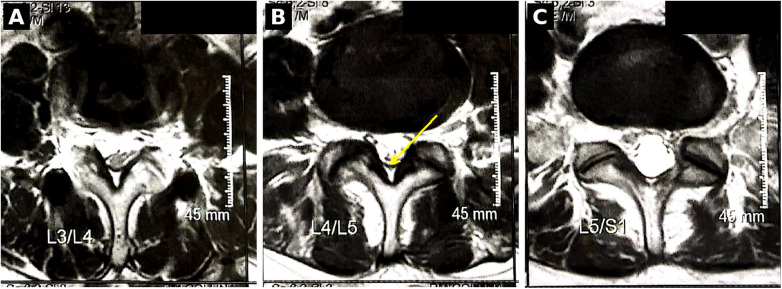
Axial T2-weighted lumbar MRI obtained after the procedure (21 April 2026) at the operated and adjacent lower lumbar levels. (A) L3–L4; (B) L4–L5, showing degenerative central canal narrowing (arrow) with facet hypertrophy and ligamentum flavum thickening (cross-sectional area approximately 119 mm^2^, reported as borderline and essentially unchanged compared with the prior 2024 examination); (C) L5–S1. The multilevel degenerative substrate is consistent with the persistent axial component. Patient identifiers have been removed.
